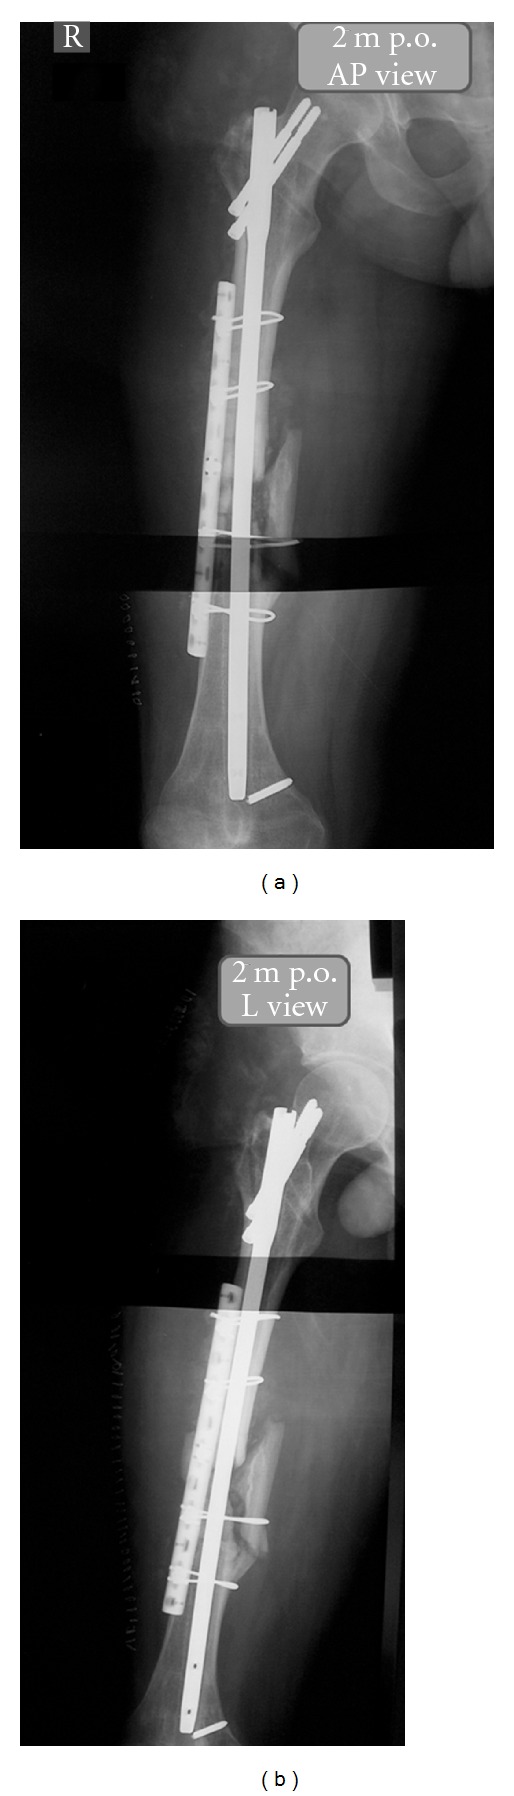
Radiographic imaging of femoral reconstruction two months postoperatively in (a) anteroposterior and (b) lateral view, showing satisfactory callus formation of both the fracture and the osteotomy of the femur.
